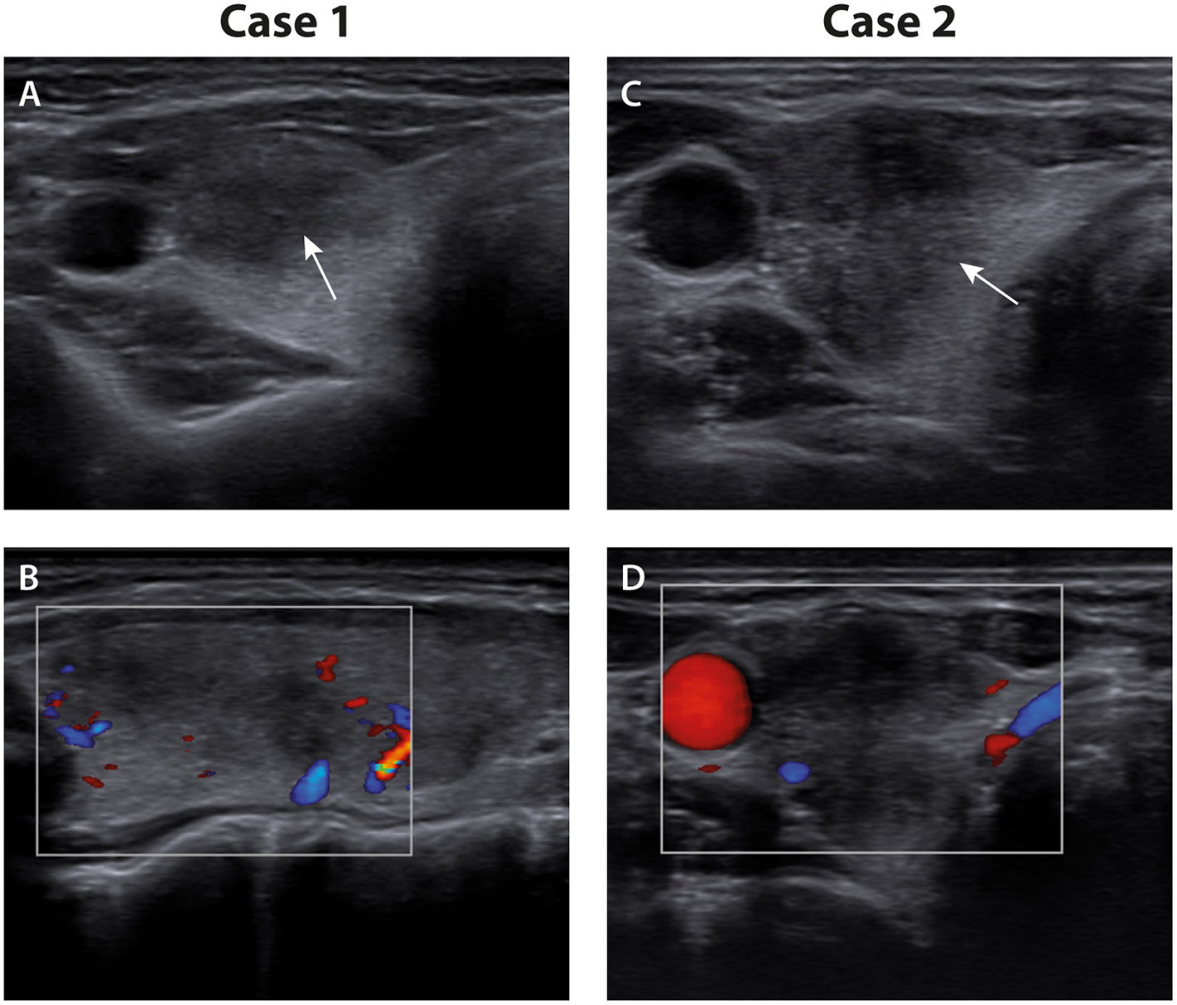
Thyroid ultrasound. (A)
